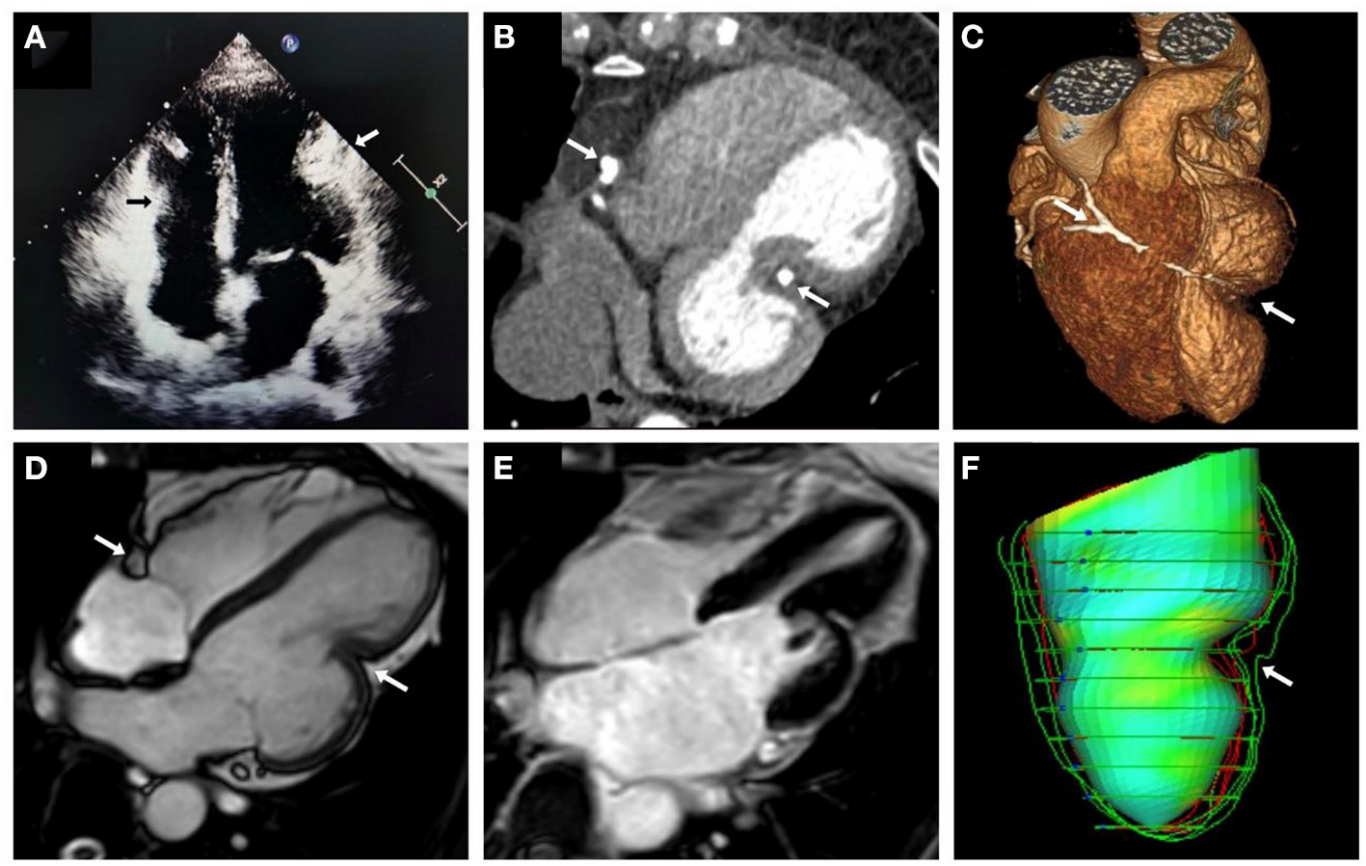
Multimodality imaging of calcific annular constrictive pericarditis. Echocardiographic in apical 4-chamber view (A), CT in 4-chambers view (B), 3D volume rendering view (C), and CMR cine imaging in 4-chambers view (D) revealed calcific annular constrictive pericarditis trapping in the right atrioventricular groove and the mid-segment of LV lateral wall (arrows) and the altered shape of “gourd-shaped” heart. CMR delayed enhanced imaging (E) showed no obvious abnormal findings. LV feature tracking model (F) also showed localized strangulation in LV and abnormal heart shape changes. CMR, cardiac magnetic resonance; CT, computed tomography; LV, left ventricle.
